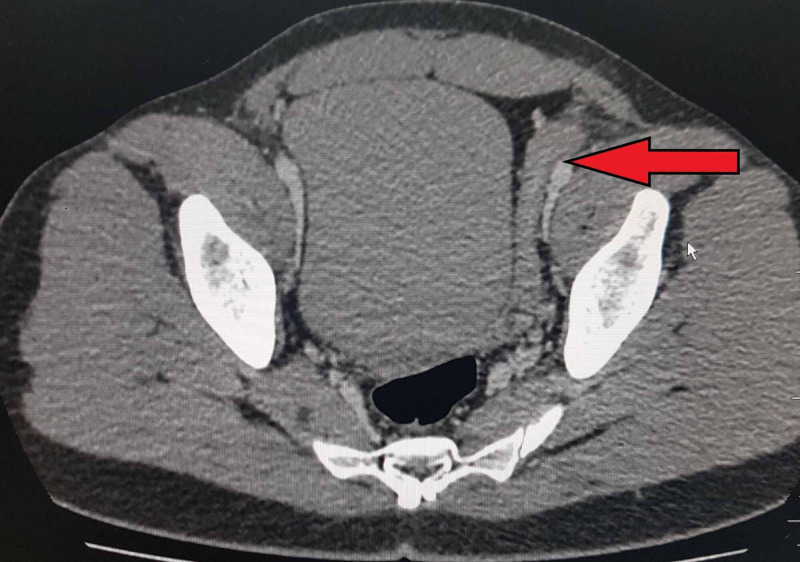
CT post-contrast showing enhanced left spermatic duct.
